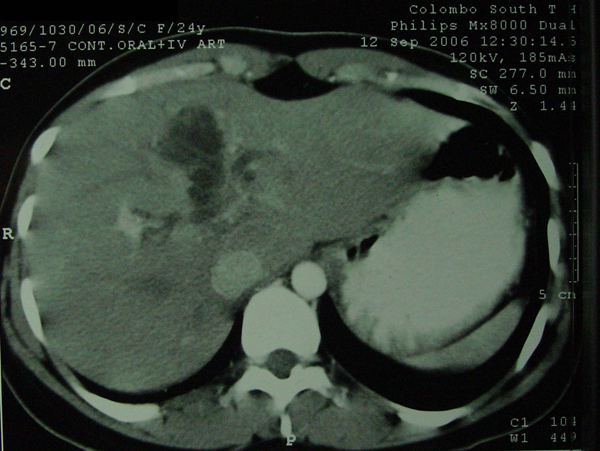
Pre-operative contrast enhanced computed tomography of the abdomen performed 2 months before surgery, demonstrating the septate intrahepatic biliary cystadenoma with extension to the left hepatic duct.
